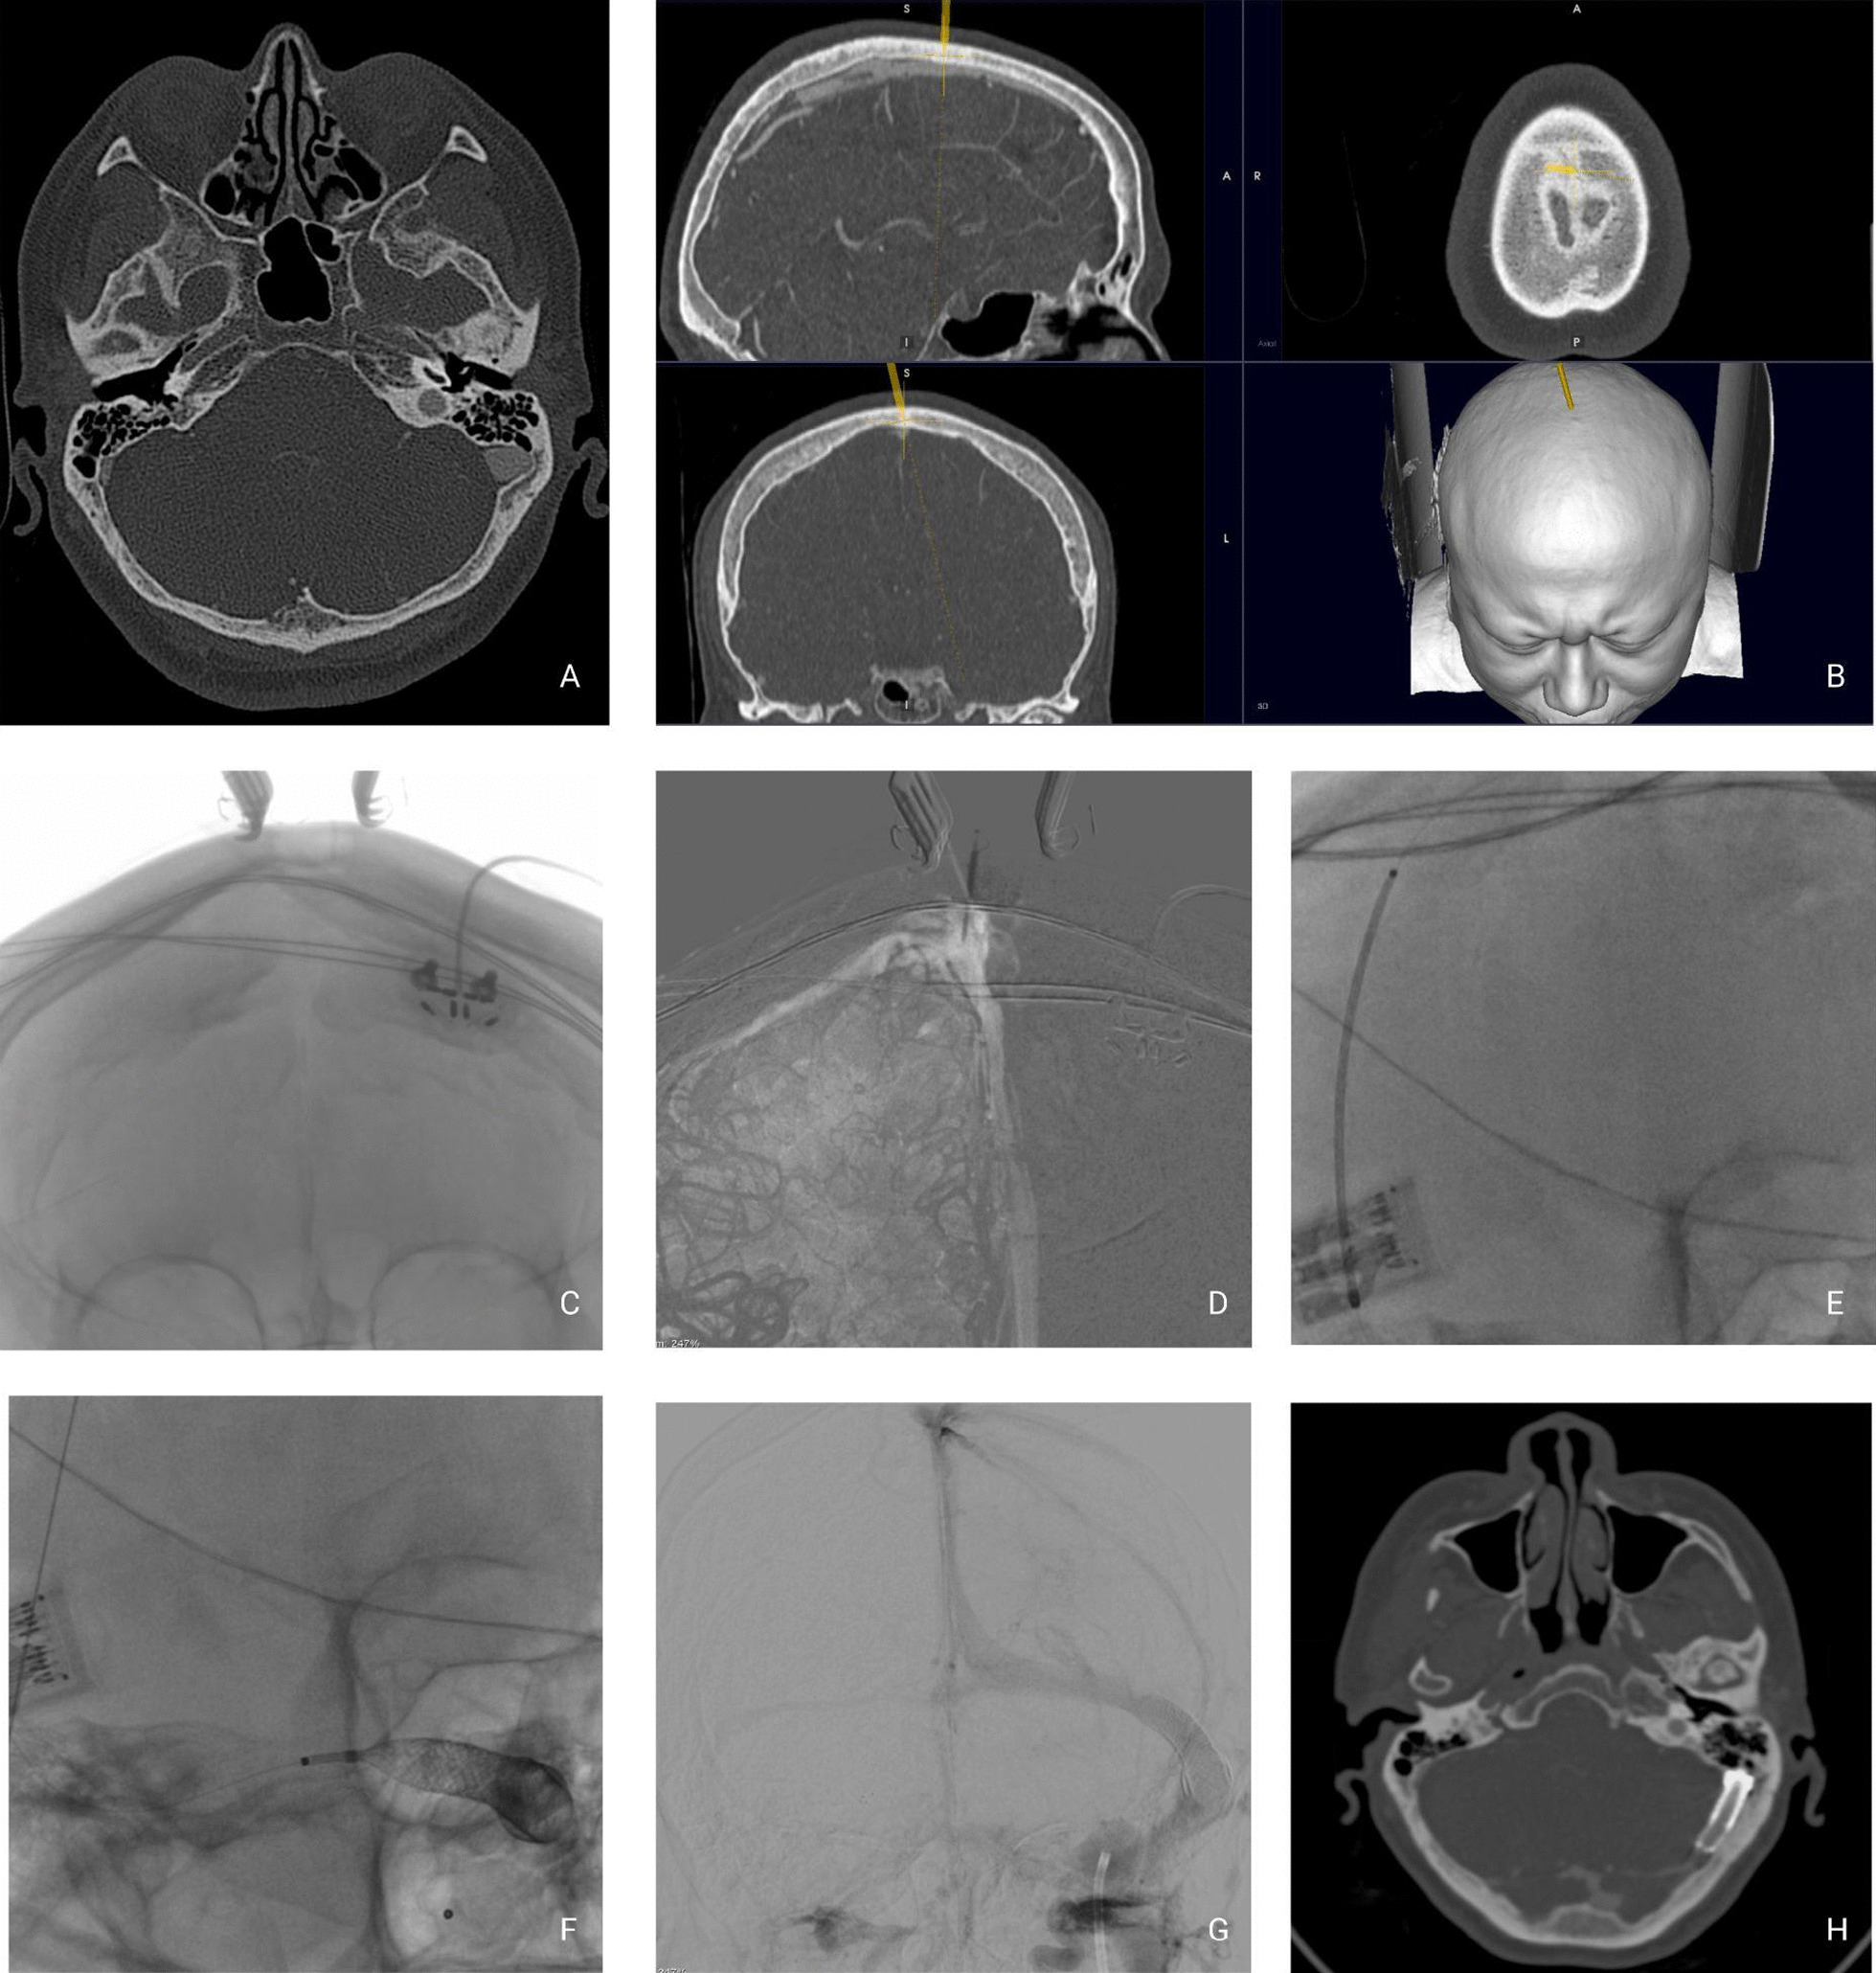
Case 1 imaging studies. A Preoperative CTA. B Neuronavigation of the SS. C–E Transoperative imaging studies of the wire advancing through the cerebral venous system to the left SS. F, G Stent deployment in the left SS lumen. H Postoperative CTA image showing the stent deployed in the left SS lumen
